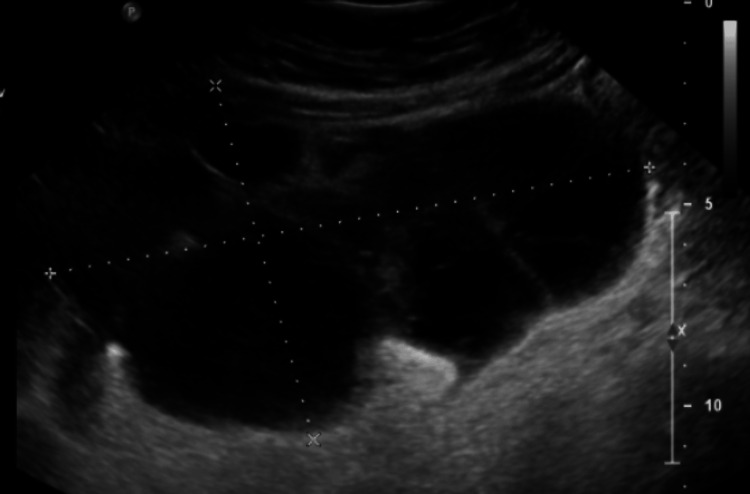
Longitudinal ultrasound of the right lower quadrant showing the septations and solid components of the adnexal mass measuring up to 15.4 cm in length. The cystic lesion appears to be associated with the ascending colon displacing loops of the small bowel.
